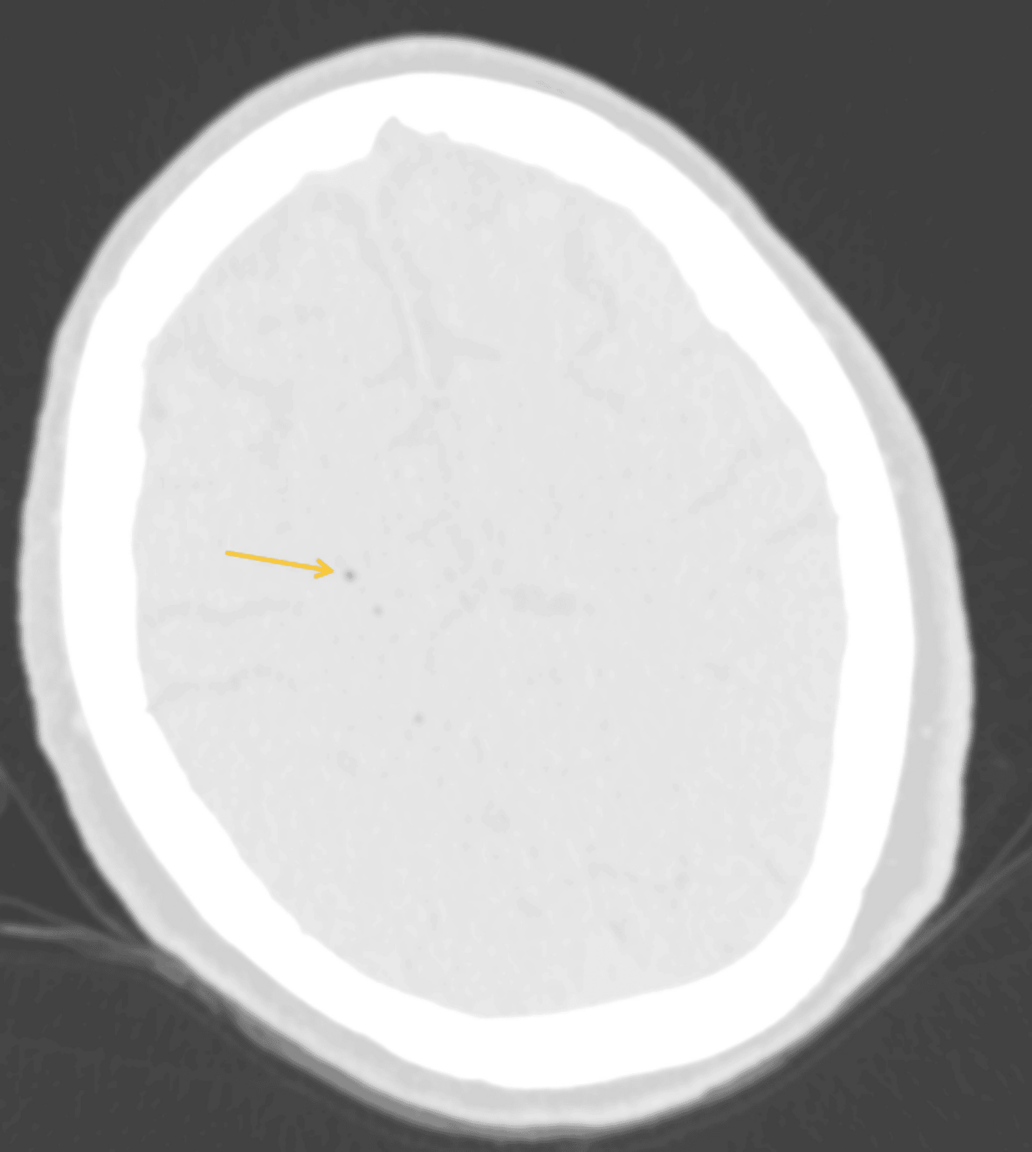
CT of the head axial non-contrast on lung windows allows for better visualization of the gas locules. CT: computed tomography
